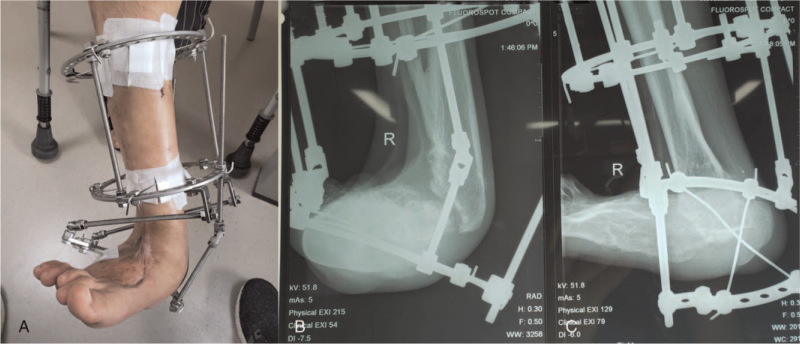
Appearance and X-ray images after the first-stage operation. (A) The ankle joint was in situ fixed by Ilizarov annular external fixation system. A contraction adjusting rod was connected to the medial part of the semicircular brace. (B and C) The semicircular brace was connected to the calcaneus by 2 cross Kirschner wire.
